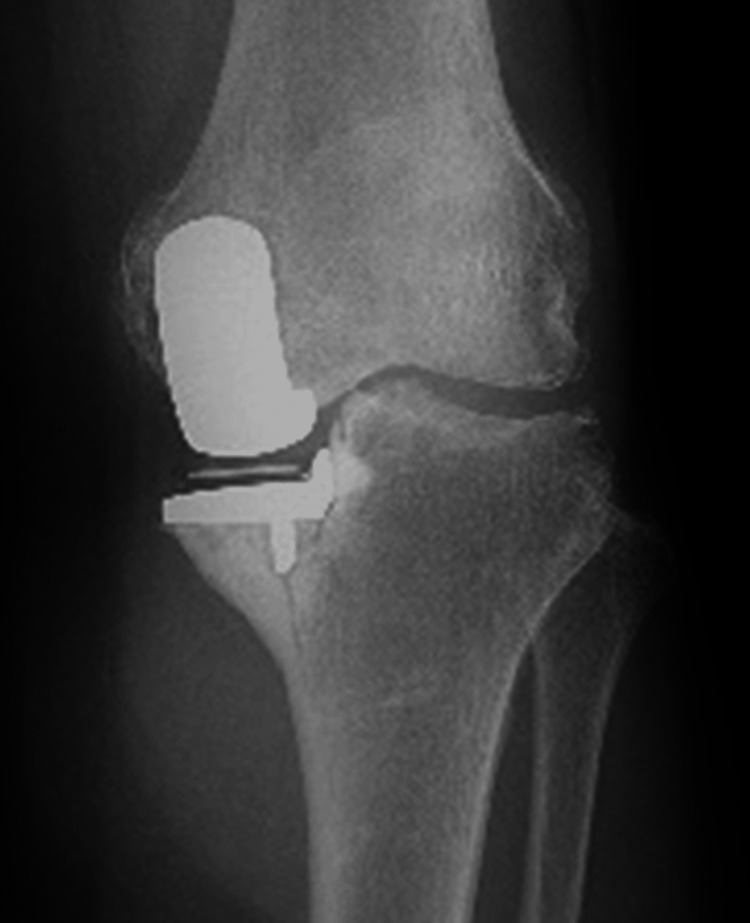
Radiography of refracture after plate removal (AP view)
